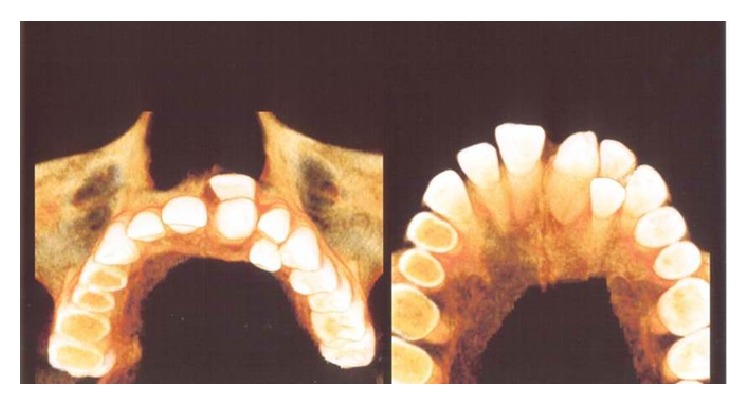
CBCT three-dimensional occlusal views depicting dilated odontoma and impacted central incisor.
